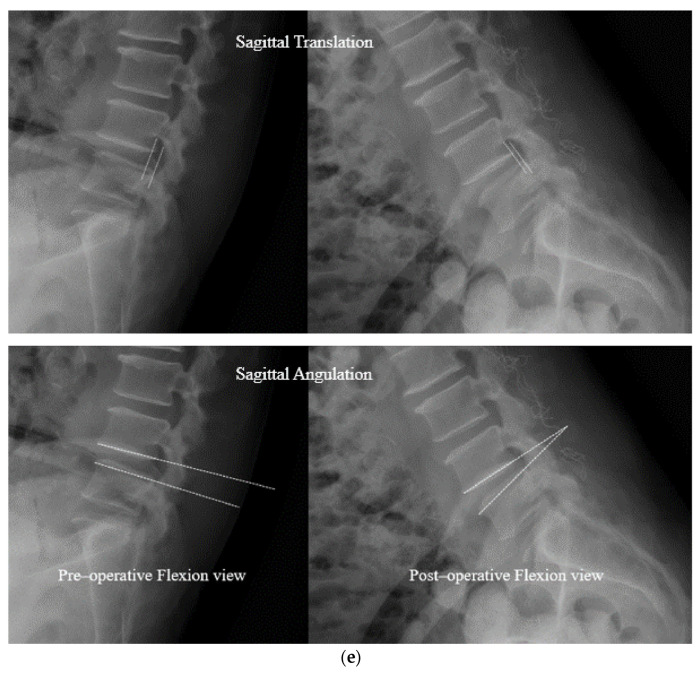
Case 2. A 79-year-old male patient suffered from low back pain and neurogenic claudication, which became aggravated after a 10 m walk. The physical examination revealed toe gait and hop test difficulties. (a) Preoperative MRI and myelogram: spinal stenosis at L2 to L5. (b) Preoperative dynamic X-ray: instability at L4–5. (c) Postoperative MRI: well-decompressed dural sac. (d) Postoperative dynamic X-ray: instability improvement at L4–5. (e) Sagittal translation decreased from 7.4 mm to 4.1 mm at L4–5. Sagittal angulation increased from −2.4° to 10.1°. Source: own composition. The small white dot line represents an extension of the posterior wall of the vertebra body and indicates the degree of listhesis. The large white dot line is an extension of the endplate line of the vertebra body and represents the degree of angulation.
